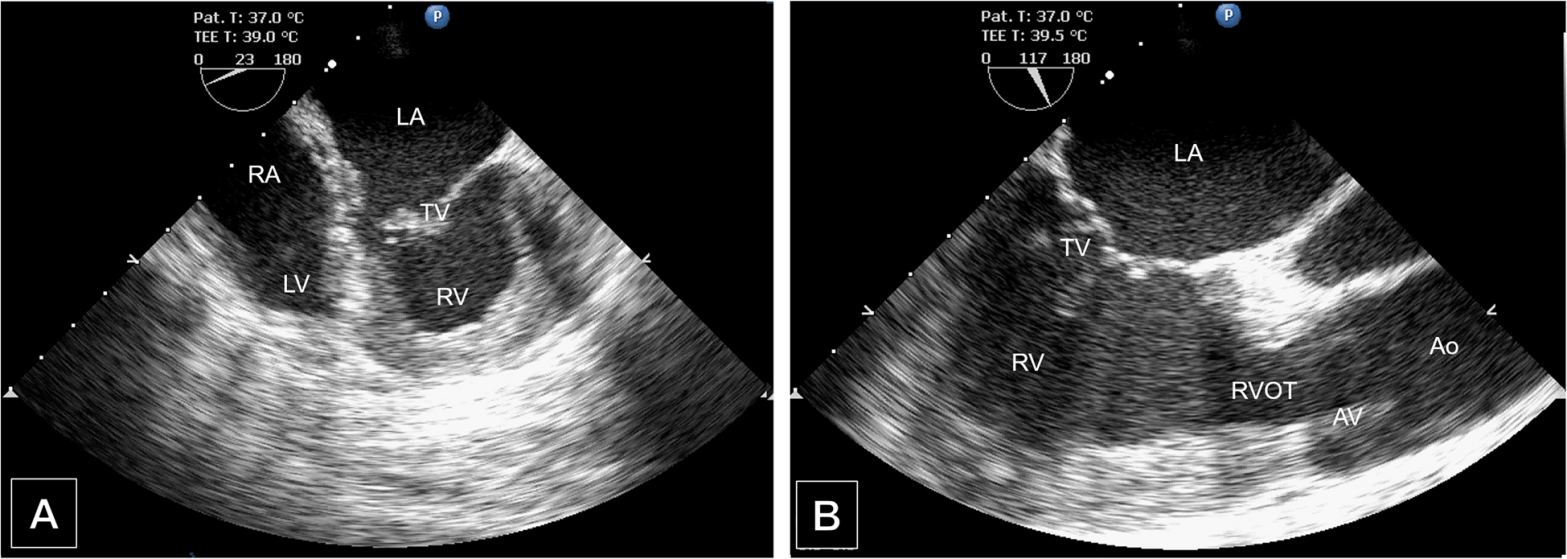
a Intraoperative transoesophageal ultrasound of the middle esophageal four-chamber view. The left atrium (LA) was associated with the morphologic right ventricle (RV) through the tricuspid valve (TV), and the right atrium (RA) was associated with the morphologic left ventricle (LV) through the mitral valve. b Intraoperative transoesophageal ultrasound of the middle oesophageal aortic valve long-axis view. The aorta (Ao) was connected to the morphologic right ventricle through the aortic valve (AV)
